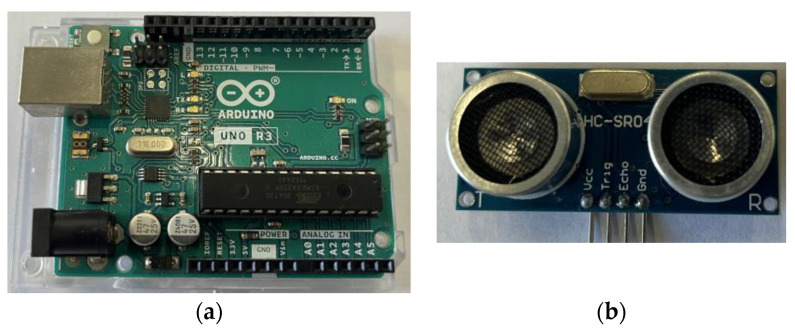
The hardware of the system. (a) Arduino UNO control board; (b) HC-SR04 ultrasonic sensor.
